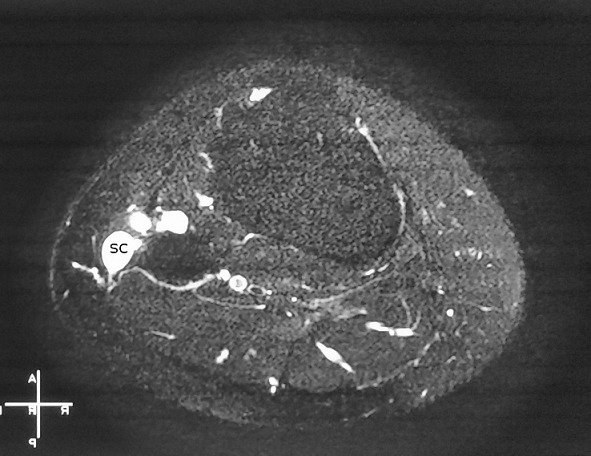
Axial view of preoperative MRI showing the multicompartmental synovial cyst (SC)
